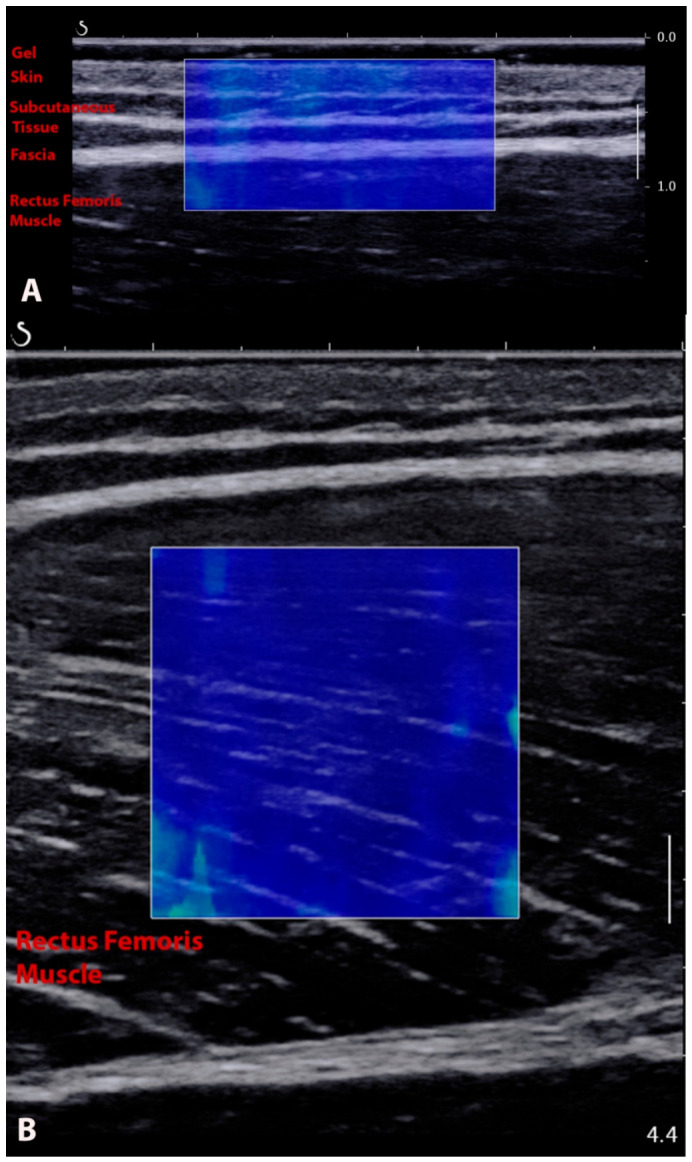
SWE scans showing skin, subQ and fascia above the RF muscle (A) and the RF muscle (B) for shear modulus measurement. The blue rectangular area represents the SWE acquisition box with the color-coded elastogram superimposed on the B-mode ultrasound image.
